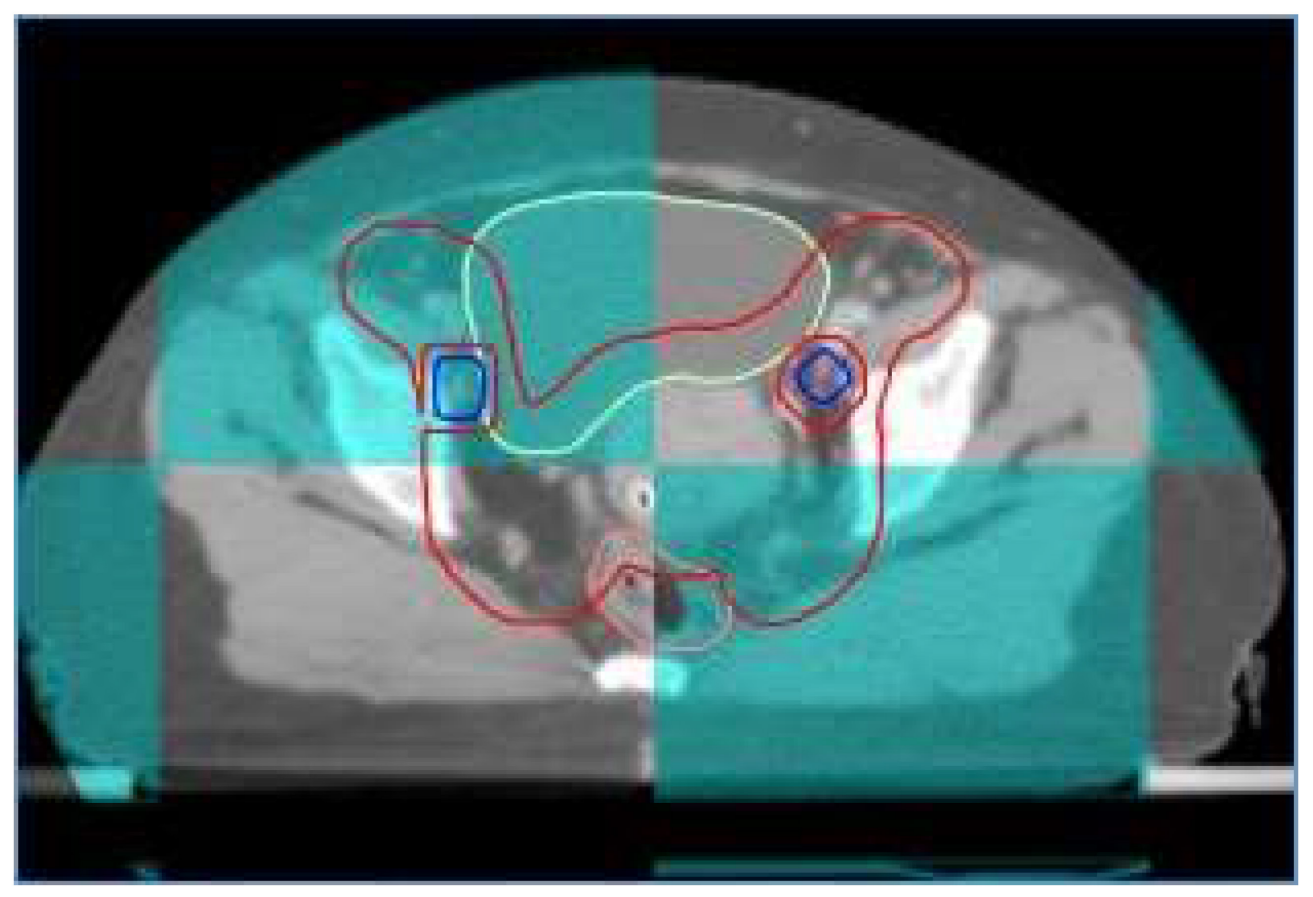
A patient with FIGO IIIC1 cervical cancer treated with IG-IMRT (Cone beam CT).
